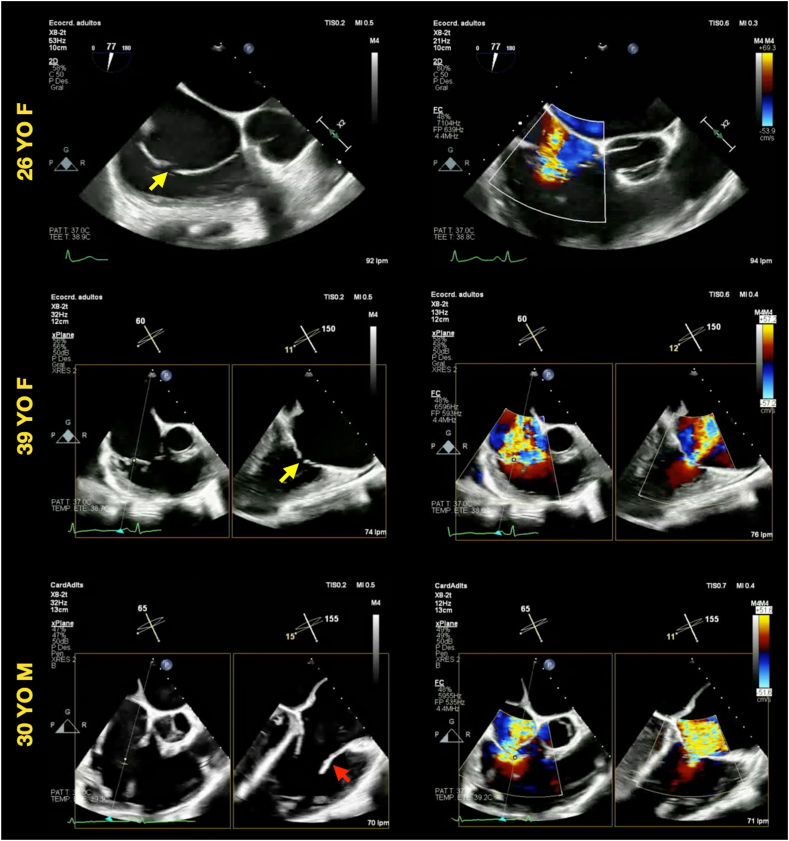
Two-dimensional morphologic assessment of the TV representative two-dimensional TEE, deep esophageal long-axis (60°-75°) view, diastolic and systolic images, without and with color-flow Doppler from 3 of the 4 patients, demonstrates the consistent findings of mild leaflet thickening (*red arrow*) and restricted systolic leaflet motion (*yellow arrows*) leading to severe TR.
